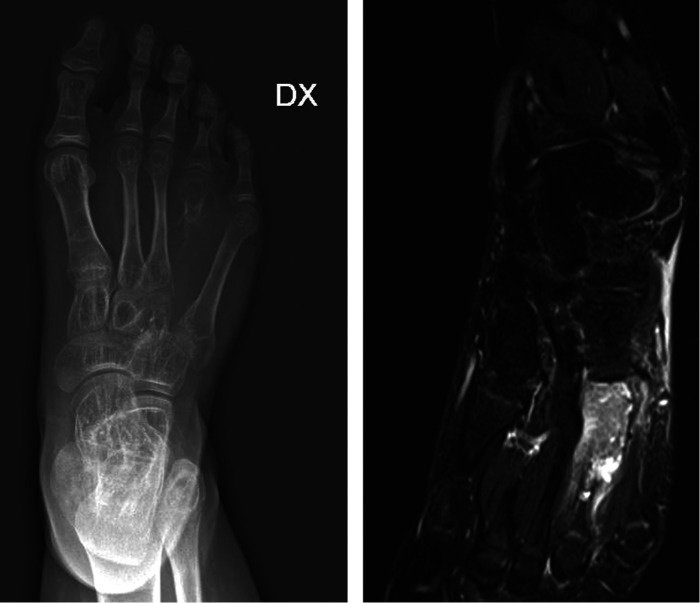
Pre-operative x-ray A/P (A) and MRI (B) view showing an expansile, lytic, encapsulated and iso-intense destructive lesion of the fourth metatarsus with soft tissue involvement.
